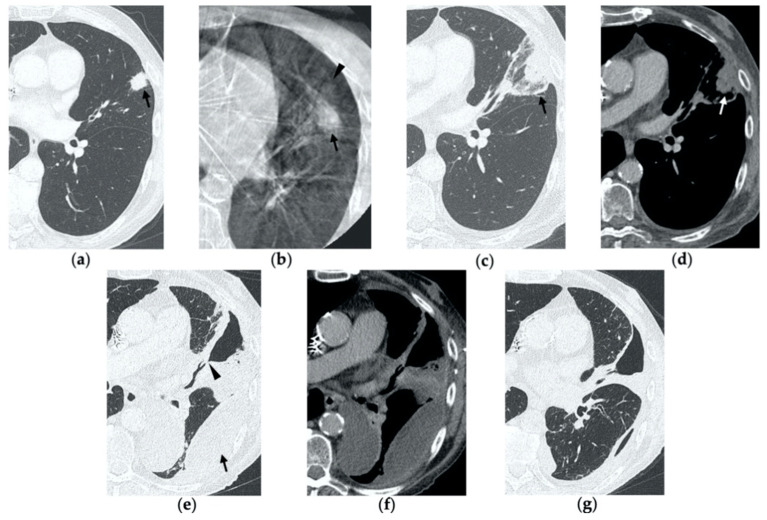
77-year-old man with biopsy proved lung adenocarcinoma in the left upper lobe. (a) Axial CT image before treatment (black arrow). (b) Cone-beam CT image obtained at the end of the procedure shows GGO (black arrow) encircling the treated lesion and a minimal pneumothorax (arrowhead), which resolved after percutaneous drainage. (c,d) On axial CT images obtained after 1 month, there is no evidence of pneumothorax and the ablation site shows a large area of increased density, with well-defined margins and a central consolidation with inner cavitation (black arrow), without contrast enhancement (white arrow). (e,f) Three-month follow-up axial CT images show a large hydropneumothorax (black arrow) near the cavitation that is clearly in communication with a bronchus (arrowhead), consistent with a broncho-pleural fistula. (g) Axial CT image of the BPF post-pleural drainage insertion.
